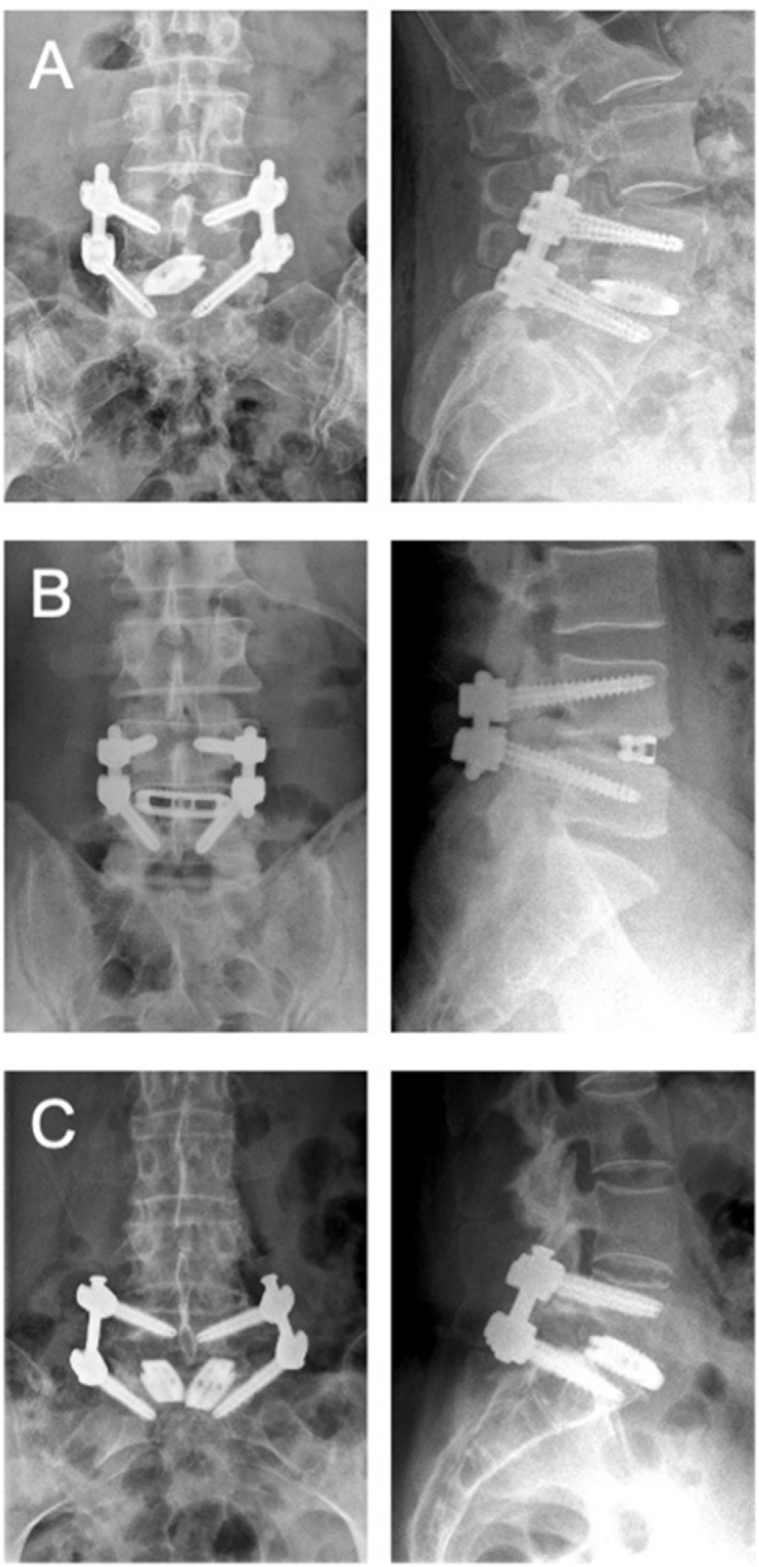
TLIF using a straight or a banana cage. Representative images (postoperative radiographs) of A TLIF with oblique insertion of a straight cage, B TLIF with a banana cage placed at the anterior edge and C TLIF using two straight cages in L5-S1.
